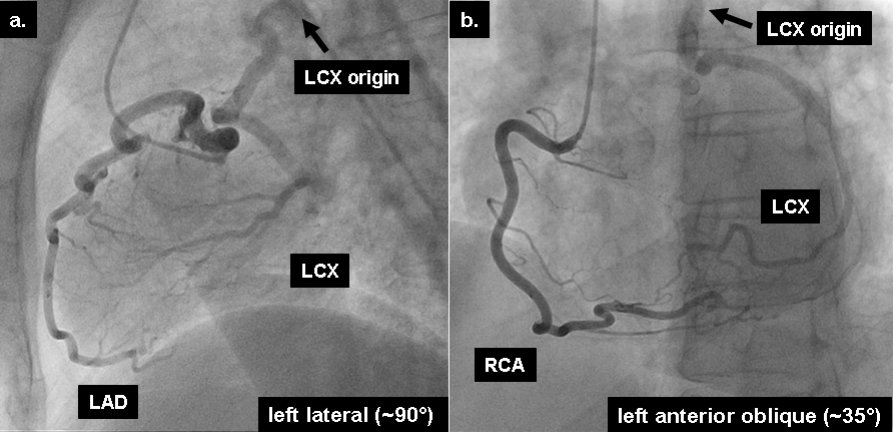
X-Ray coronary angiography shows the retrograde filling of an anomalous left circumflex artery (LCX) though collateral vessels provided by (a) the left anterior descending artery (LAD) and (b) the right coronary artery (RCA).
